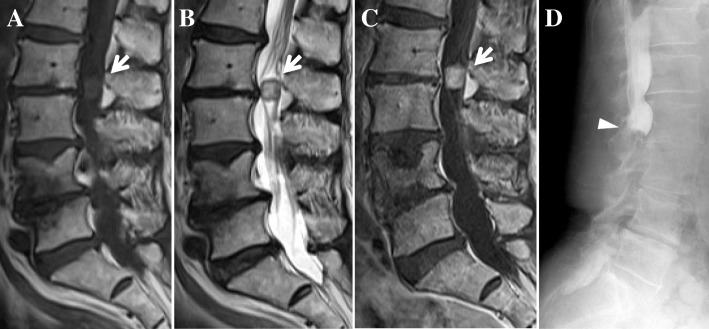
a–c Sagittal magnetic resonance imaging. a T1-weighted image. b T2-weighted image. c T1-weighted gadolinium-enhanced image. Intradural extramedullary mass at the level of L2–L3 showing T1-low, T2-high signals enhanced uniformly with gadolinium (*arrow*). d Lateral myelography showing a total block of contrast below the level of L2–L3 and capping of contrast by the mass (*arrowhead*)
